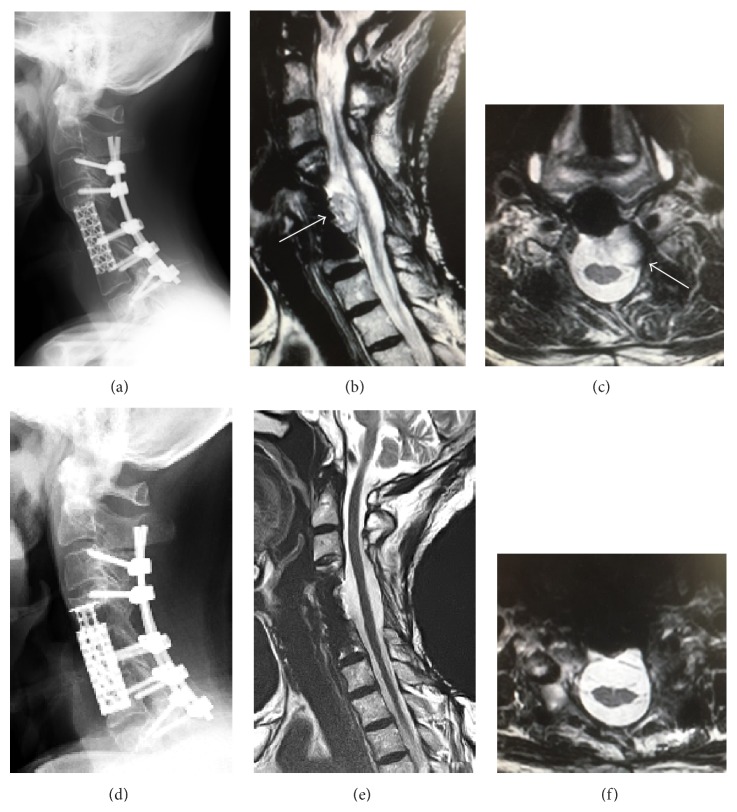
Roentgenogram after surgery. (a) Lateral radiograph showing posterior cervical interbody fusion with the artificial vertebral body of C3 to T1 after the first surgery. (b, c) Sagittal (b) and axial MRI scan (c) at 6 years after the initial surgery with a third recurrence of the tumor. (d) Lateral radiograph of the cervical spine, and sagittal (e) and axial views (f) of the MRI image, showing no recurrence at 8 years 4 months after the first surgery.
